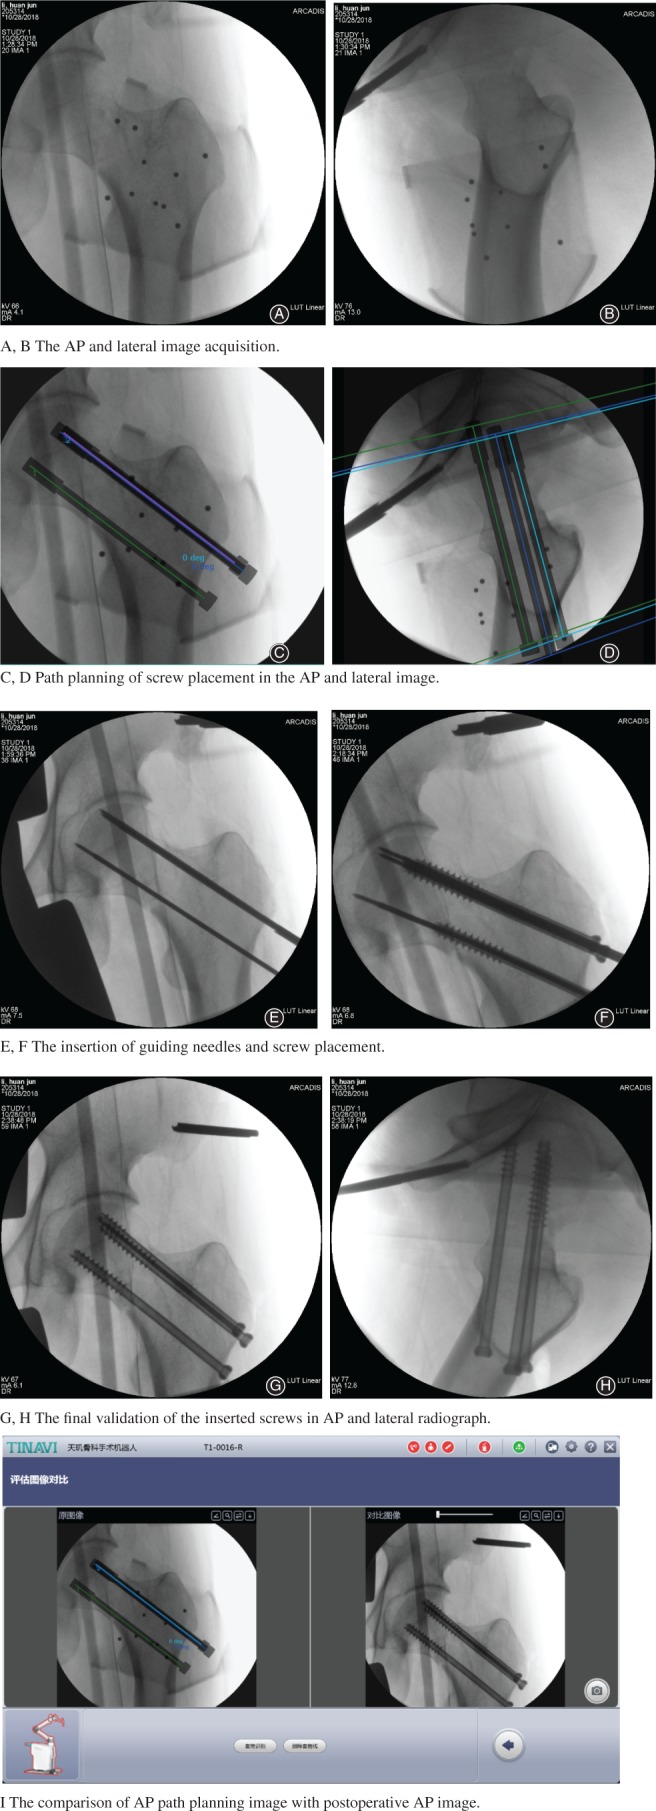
A 45‐year‐old man with a left femoral neck fracture. The surgical procedure of TiRobot‐assisted percutaneous cannulated screw fixation. (A, B) The anteroposterior (AP) and lateral image acquisition. (C, D) Path planning of screw placement in the AP and lateral image. (E, F) The insertion of guiding needles and screw placement. (G, H) The final validation of the inserted screws in AP and lateral radiograph. (I) Comparison of AP path planning image with postoperative AP image.
